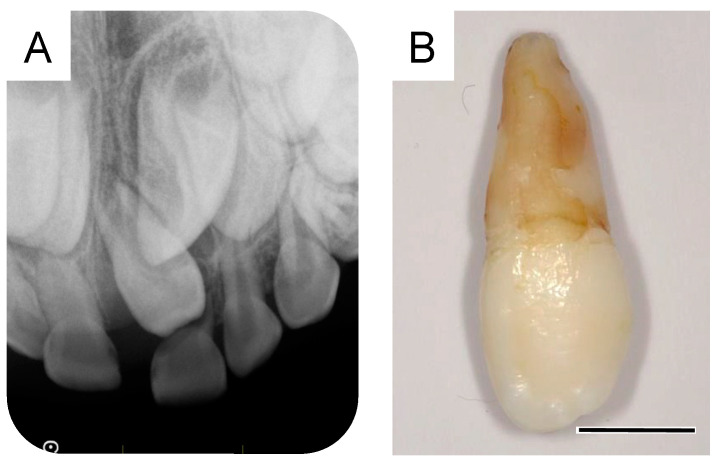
Extraction of the first supernumerary tooth. (A) Periapical radiograph of the first supernumerary tooth detected at the age of 5 years and 5 months. (B) Extracted first supernumerary tooth at the age of 5 years and 11 months. Scale bar: 5 mm.
